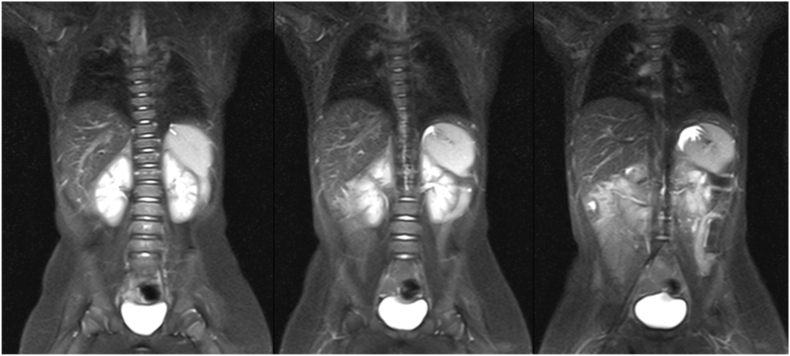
Sequential coronal MRI images of continuous splenogonadal fusion, demonstrating the tail-like structure connecting the normally located spleen and left ovary.
